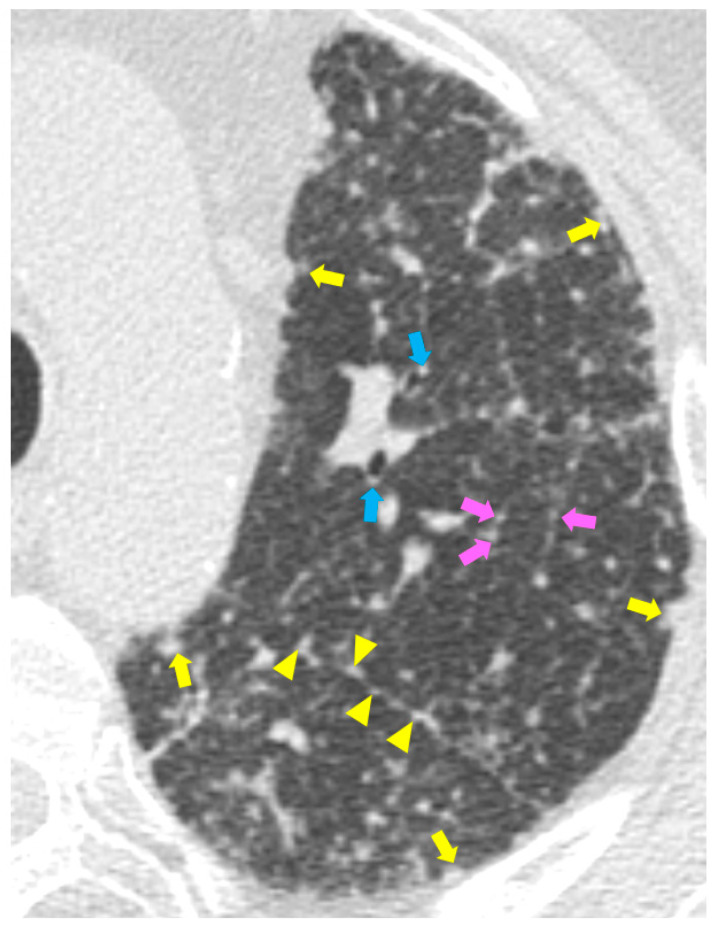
Computed tomography (CT) typical findings of sarcoidosis. The axial HRCT scan of the left lung in a patient with pulmonary sarcoidosis shows the typical perilymphatic distribution of micronodules along the peribronchovascular (blue arrows), subpleural interstitial space (yellow arrows), and interlobular septa (pink arrows). The yellow arrowheads show the typical subpleural distribution of the micronodules along the fissure.
